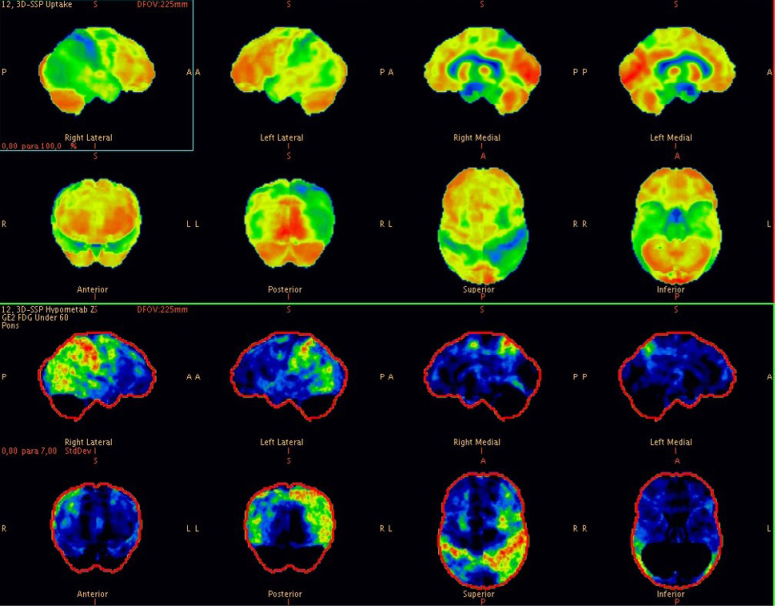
Metabolic map of brain Positron Emission Tomography with [18F]fluordeoxyglucose (FDG PET) study of case 2 revealing significant asymmetric, right-predominant glycolytic hypometabolism affecting posterior parietal regions and precuneus.
